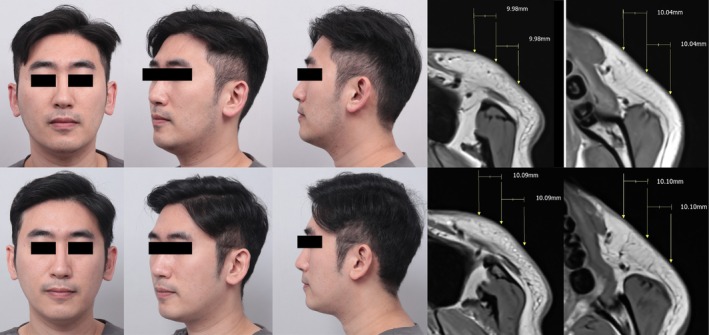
Clinical photograph and T1‐weighted MRI of pre‐treatment (upper) and post‐3 month (lower) with microfocused ultrasound.
